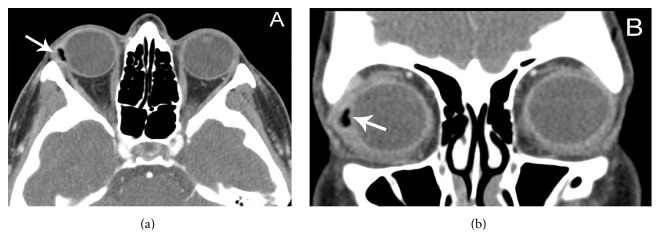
(a) Axial CT image identifying a hypoattenuated lesion with slight localized mass effect on the globe with surrounding soft tissue attenuation. Note the irregular, jagged borders of the lesion. (b) Coronal cut shows more definitive surrounding soft tissue attenuation. The hypoattenuation mass, which was confirmed by surgical explantation to be a retained silicone sponge element, was noted to have an attenuation of −284 Hounsfield units.
